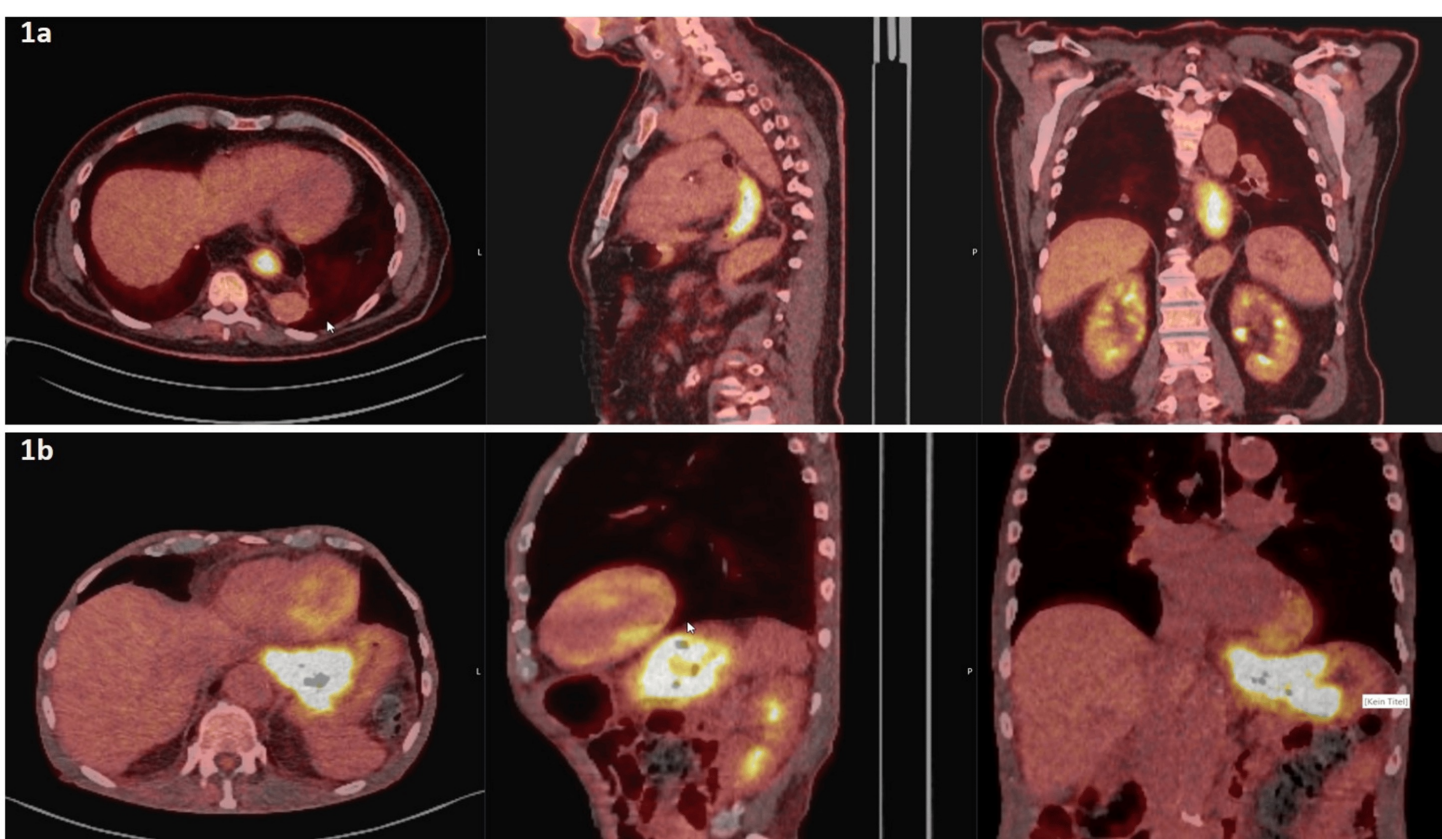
Primary tumor location and extent on FDG-PET/CT for Patient 1 (1a) and Patient 2 (1b) FDG-PET/CT: Fluorodeoxyglucose-positron emission tomography/computed tomography
